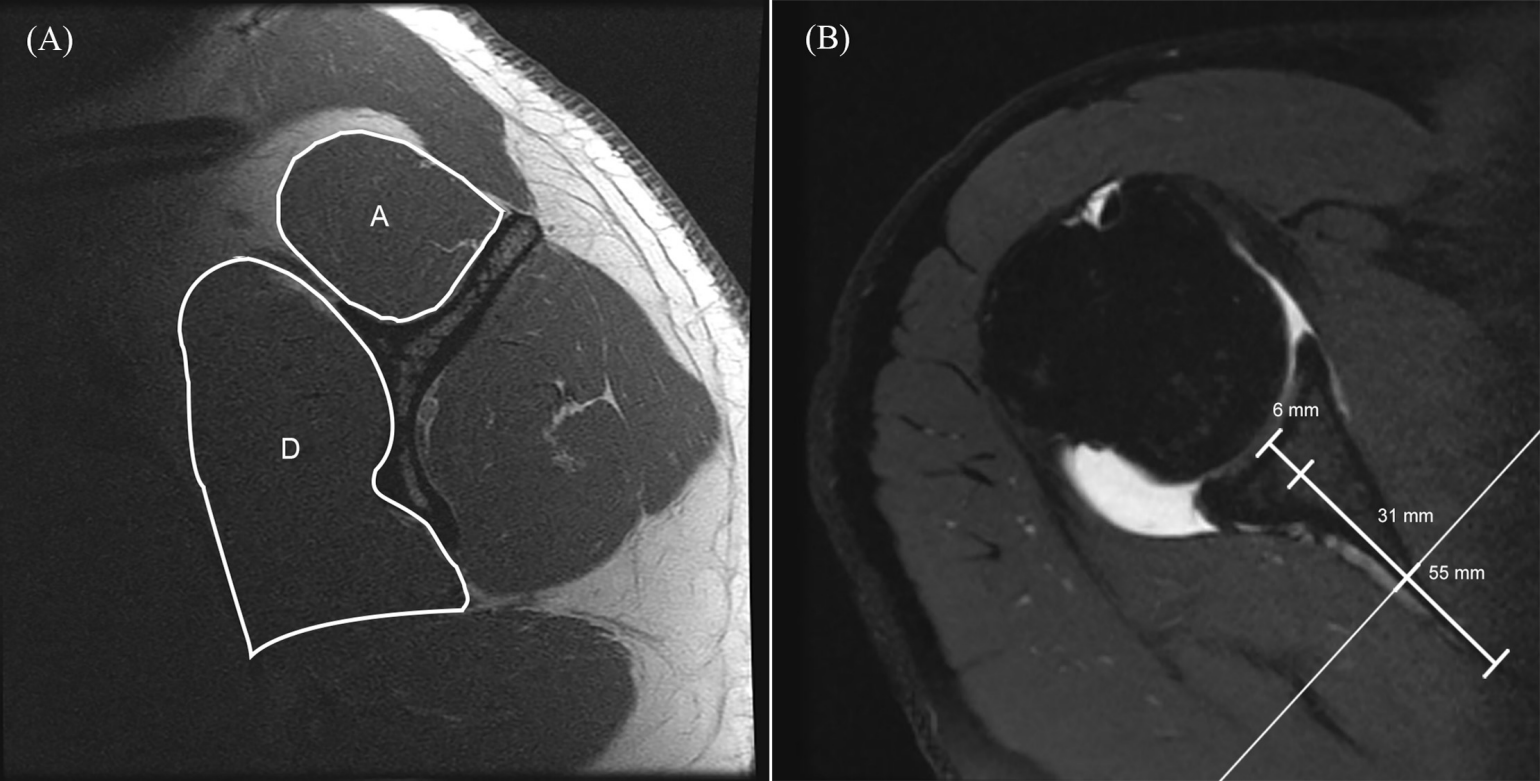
Measurement example for “set position”. According to Yanagisawa et al. [19] the cross-sectional area (CSA) measurement of the rotator cuff muscles was also performed at more medial slices. Left panel shows a sagittal T1-weighted MR-arthrography image of the same patient (see Figs 2 and 4) and highlights the CSA measurement of the supraspinatus (955 mm^2^, A) and subscapularis (2306 mm^2^, D) 31 mm medial to the center of the glenoid cavity. The right panel, which shows an axial PD-weighted image, indicates the suggested measurement levels for males as the medial distance from the glenoid cavity base: 6 mm for the CSA measurement of the teres minor, 31 mm for supraspinatus and subscapularis (see reference line) and 55 mm for infraspinatus.
